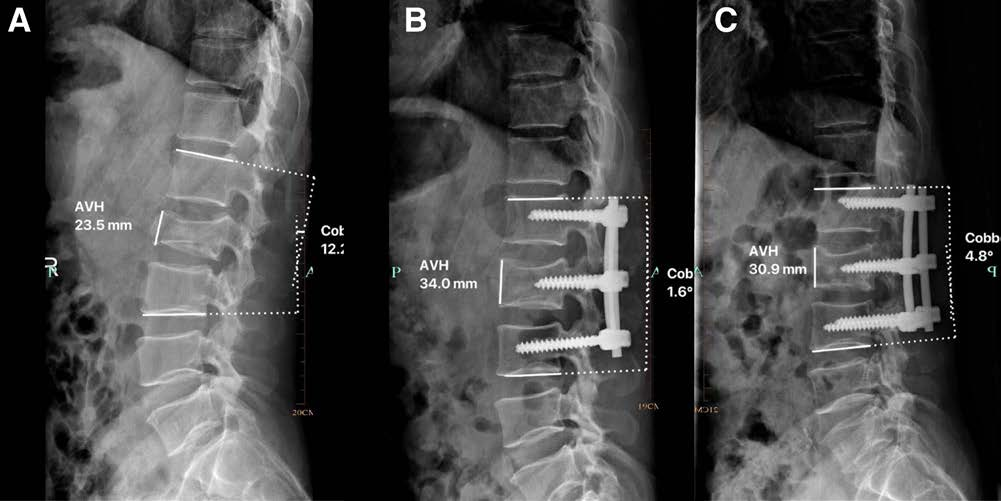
Pre-operative (A), post-operative (B) and follow-up (C) radiological measurements on a lateral radiograph, including anterior body height and Cobb angle.
